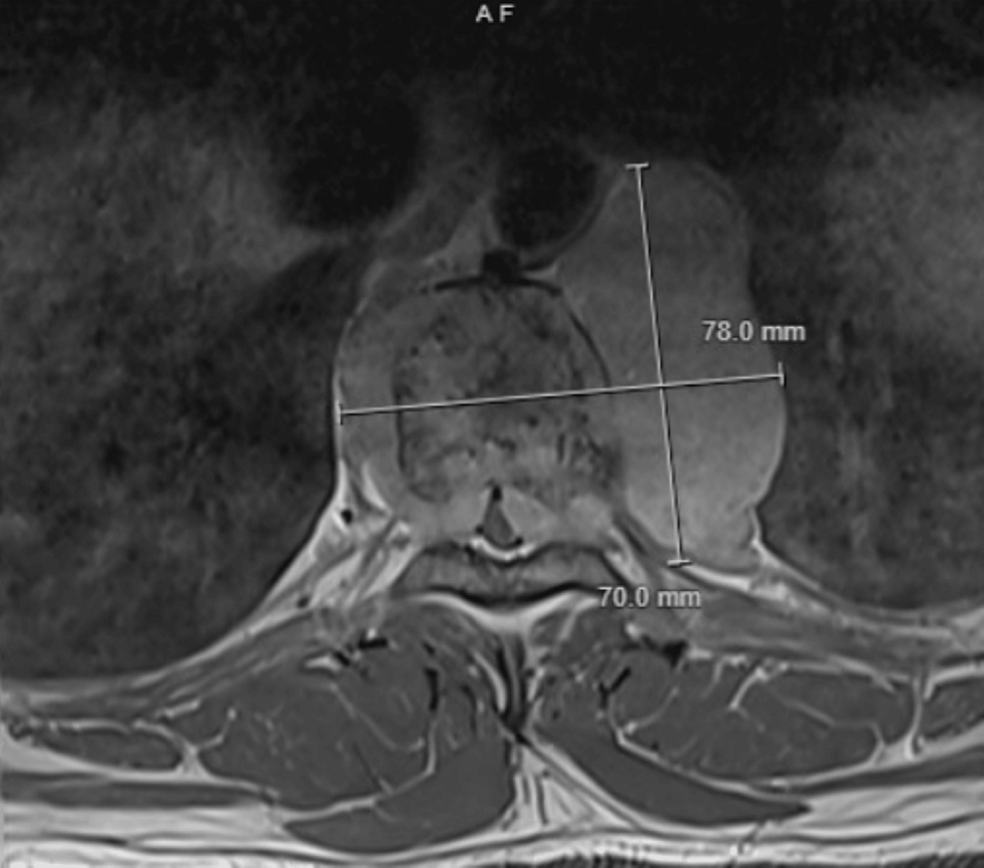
T1 weighted axial thoracic MRI revealing a large soft tissue and osseous mass centered at T8 vertebral body with large paravertebral extension more toward the left as well as significant ventral epidural and foraminal mass extension at T8-T9. MRI: magnetic resonance imaging
